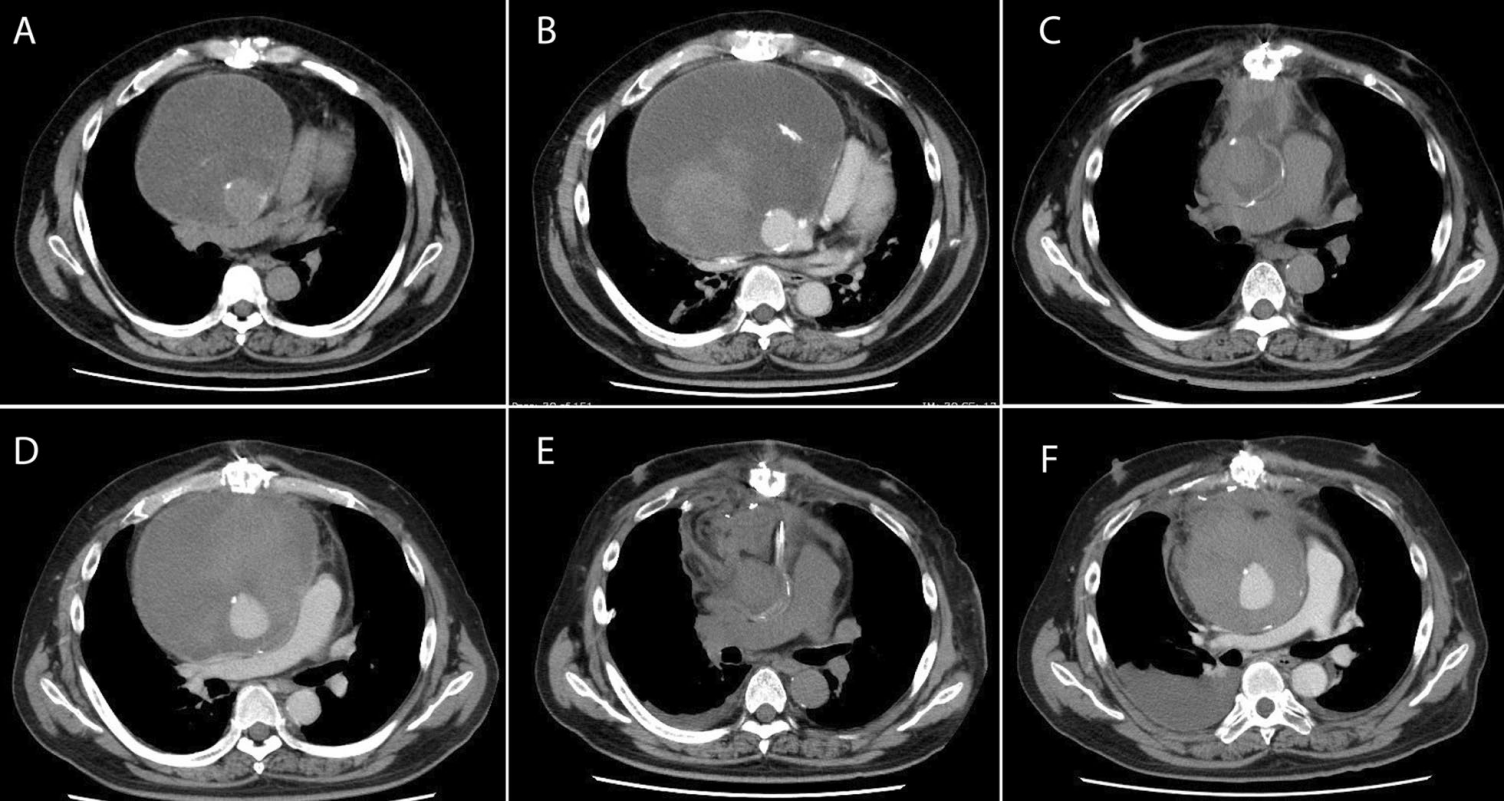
Findings on CT scans from years 6 to 9 after the initial surgery. a POY 6. A mass was detected around the ascending aorta. b POY 7. A 100-mm mass was detected around the ascending aorta and the ascending aortic graft was replaced. c A CT scan obtained immediately thereafter showed slight effusion around the ascending aorta. d Two months later, fluid retention was again observed around the ascending aorta. e The hematoma capsule was removed, and after confirming hemostasis, the left vastus lateralis muscle was filled into the anterior mediastinum. f Six months later, further hematoma was found in the mediastinum. CT, computed tomography; POY, postoperative year
